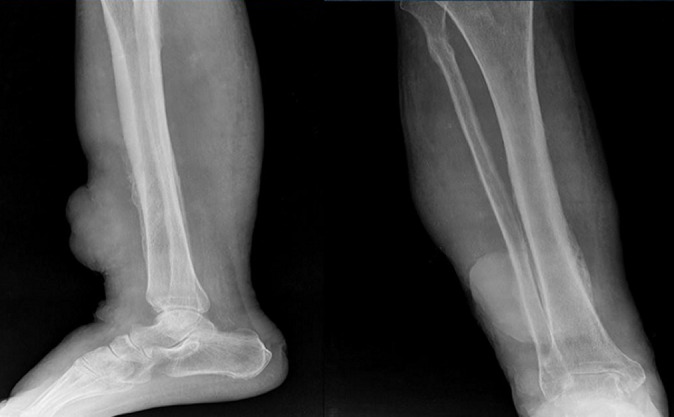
X-ray anteroposterior and lateral projection of the right leg shows a lobulated soft tissue swelling in the anterior aspect of the lower one third of the leg with few calcific areas within and associated periosteal reaction the adjacent bones of the leg
